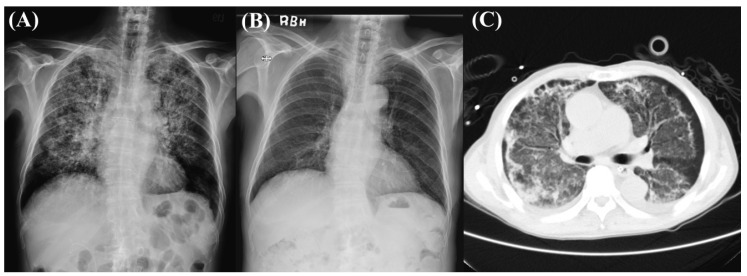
(A) Chest X-ray (CXR) before intubation: bilateral asymmetric patches of hazy opacity, relatively sparing lateral lung bases. (B) CXR two weeks after extubation: great resolution of bilateral infiltration and relatively clear lung fields. (C) Chest computed tomography showed diffuse ground glass opacities mixed with patchy consolidation, predominantly in upper and middle lung zones, with subpleural sparing.
